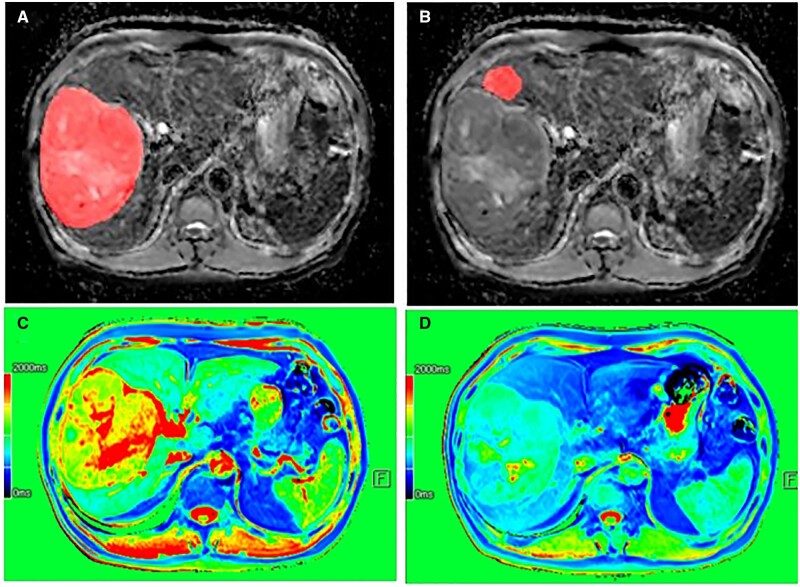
The region of interest in the tumoral and peritumoral areas. (A) Tumor delineation (marked area) on the ADC MR image; (B) peritumoral area delineation (marked area) on the ADC MR image (an area of diameter 2 cm, avoiding blood vessels and bile ducts is selected); (C) T1-mapping image before contrast injection (T1 value is 1,515.59 ms before contrast injection); (D) T1-mapping after contrast injection (T1 value is 1,156.65 ms after contrast injection). ADC = apparent diffusion coefficient, MR = magnetic resonance.
